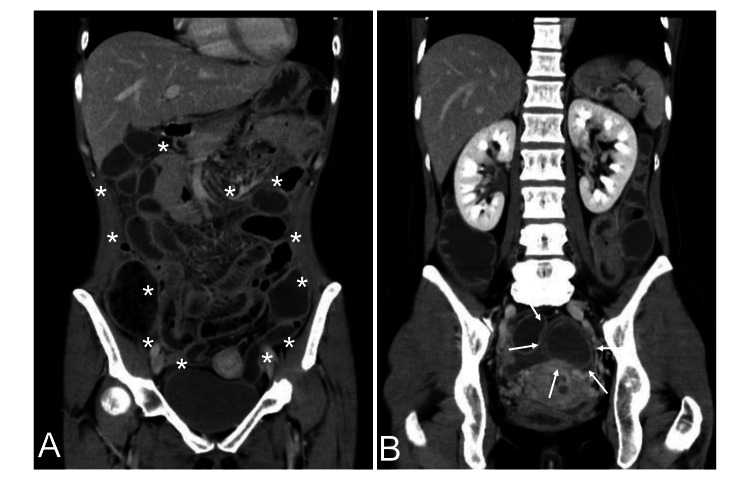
Previously healthy 44-year-old woman entered the general ward of another hospital complaining of lower abdominal pain and nausea. Abdominal computed tomography showing a thickened wall of the entire small intestine (A; asterisk) and ascites in the Douglas fossa (B; arrow).
